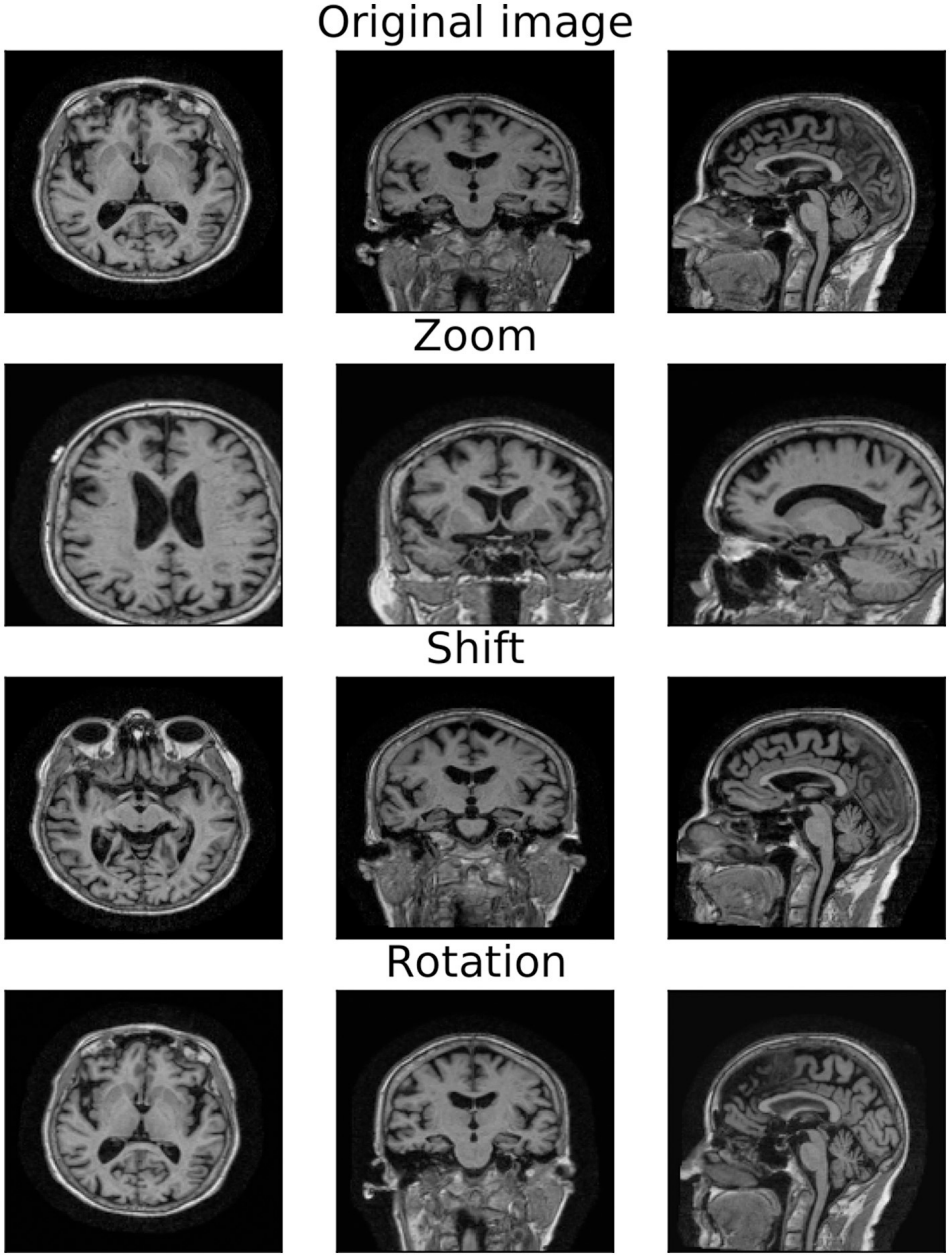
Original and transformed MRI image. 2D projections of the original MRI image (first row) and the augmented image obtained by applying *zoom*
